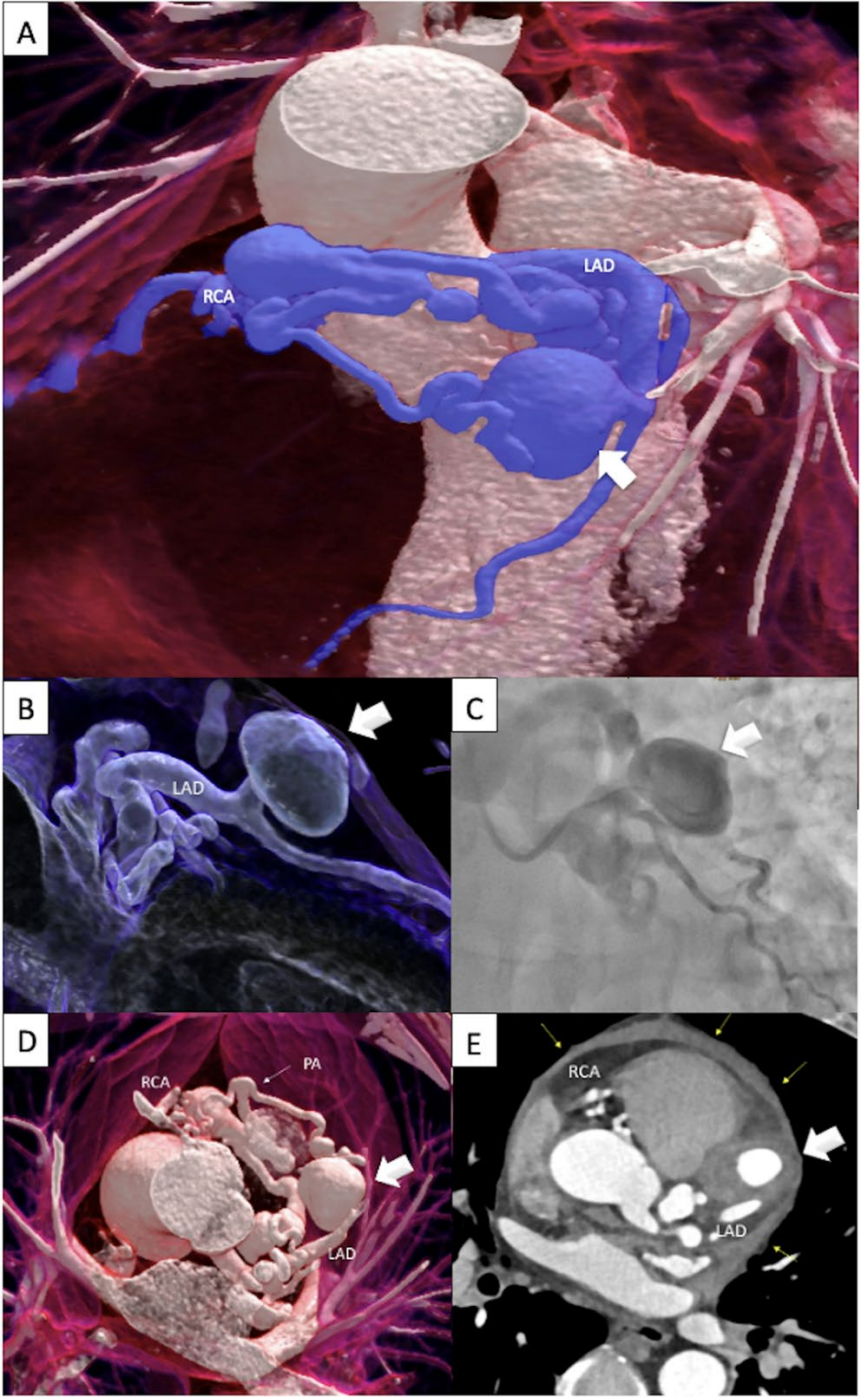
Ruptured Coronary Aneurysm (LAD). Panel A-B: Coronary CTA: 3D volume-rendered technique (VRT) image demonstrating the coronary fistula originating from the LAD with an associated large 4 cm size aneurysm (white arrow), connecting to the pulmonary artery (PA) and RCA. Panel C: Invasive coronary angiography depicting a large LAD aneurysm without evidence of active bleeding. Panel D. Coronary CTA 3DVRT: white arrow pointing at the LAD aneurysm. Panel E. Axial multiplanar reconstruction (MPR) showing an inhomogeneous wall thrombus within the LAD aneurysm, with CT attenuation values up to 69 HU, with direct attachment to the pericardium. The pericardial effusion (yellow arrows) demonstrated higher attenuation (60 HU) in the basal regions, while CT densities of the effusion in the mid-section and more cranially were consistency lower (30 HU). Therefore, hemorrhage into the pericardial space was supected (“sealed perforation”). The coronary artery fistula (CAF) had a large entry ostium into the pulmonary trunk, measuring 4.5 mm (white thin arrow). PA = pulmonary artery; RCA = right coronary artery; LAD = left anterior descending artery
